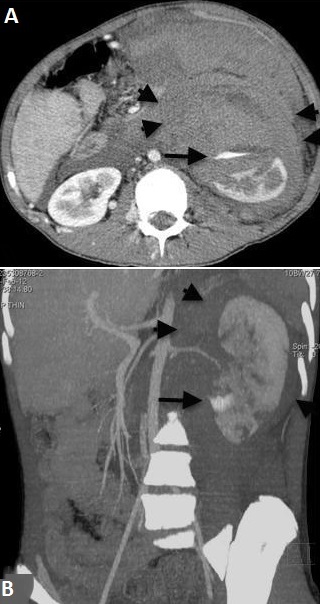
Axial (A) and 3D reconstructed coronal (B) images of the contrast-enhanced computed tomography demonstrates hematoma in the left retroperitoneal region associated with the kidney (short arrows) and signs of contrast leveling compatible with active bleeding (long arrows)
